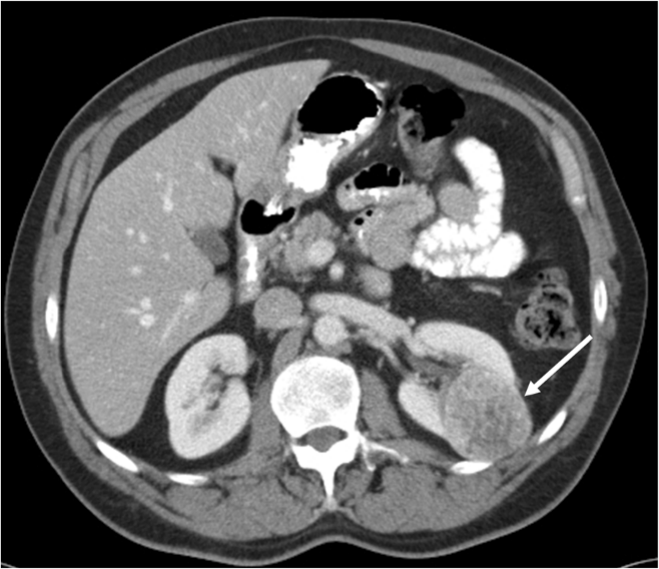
A 45-year-old male with Fuhrman grade 3 ccRCC showing well-circumscribed tumor margin. Contrast-enhanced transverse CT image demonstrate a predominantly solid and heterogeneously enhancing mass (arrow) in the mid portion of the left kidney. The tumor shows a well-circumscribed clear margin to the normal renal parenchyma.
